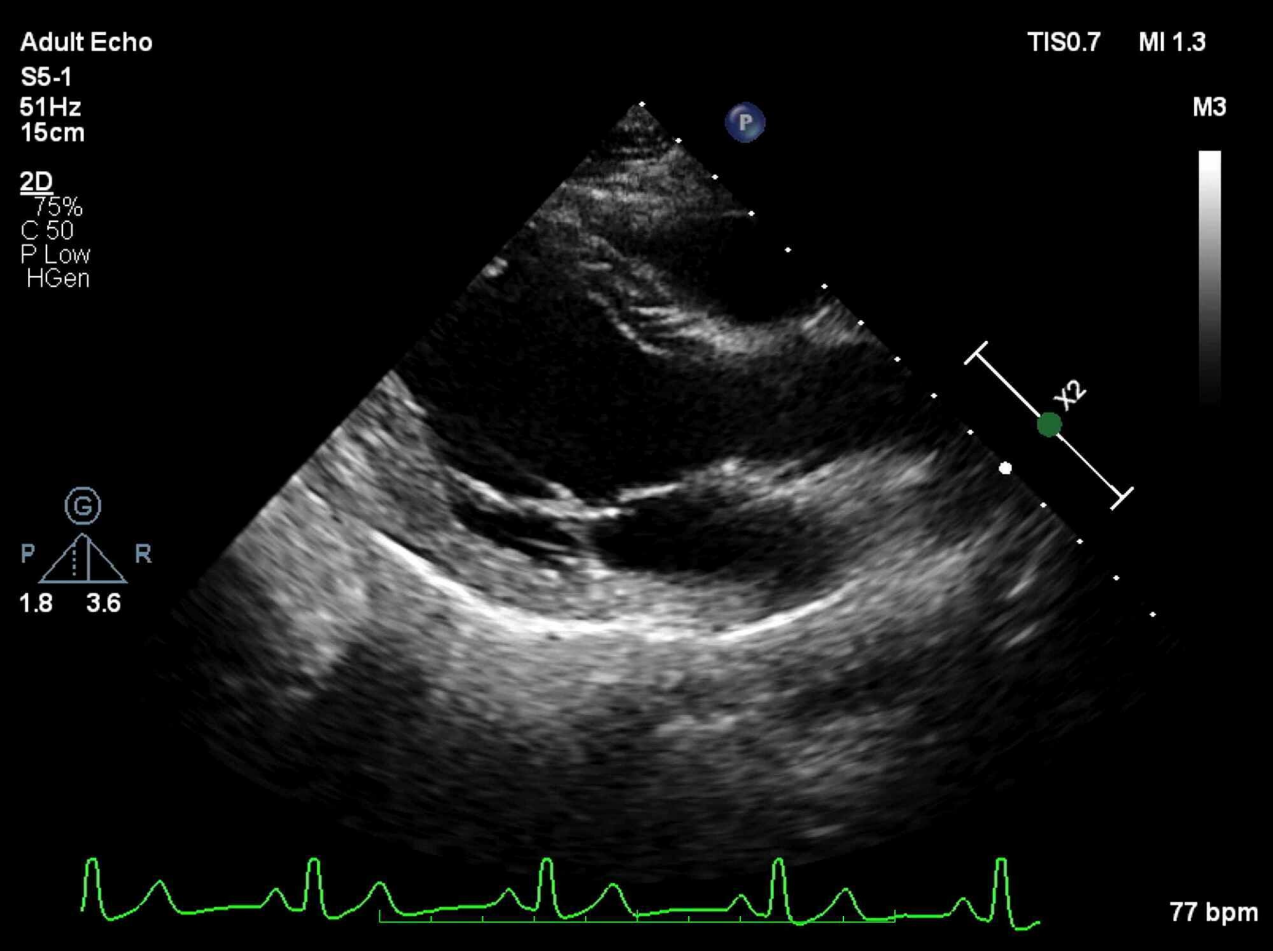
Transthoracic echocardiography at six months of follow-up. Parasternal long-axis view showing normal-sized left ventricle. The LV internal dimensions were 54 mm in diastole and 39 mm in systole. LV, left ventricular
